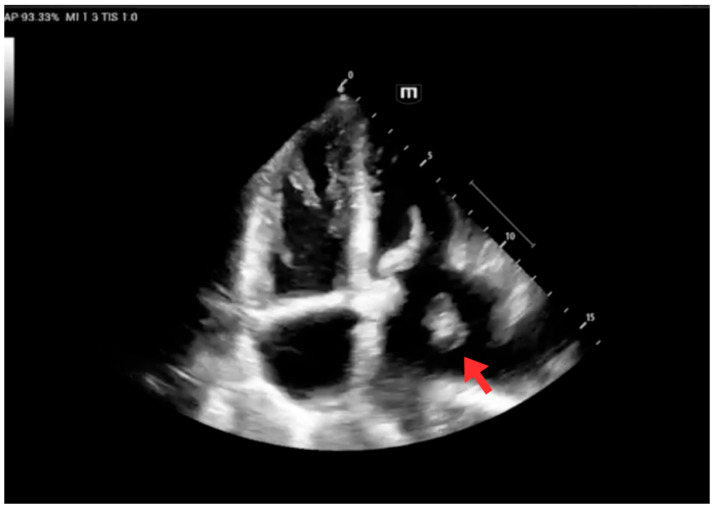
Transthoracic echocardiogram at admission. The study shows cardiac chamber dilation and a mobile thrombus in the left atrium (red arrow).
